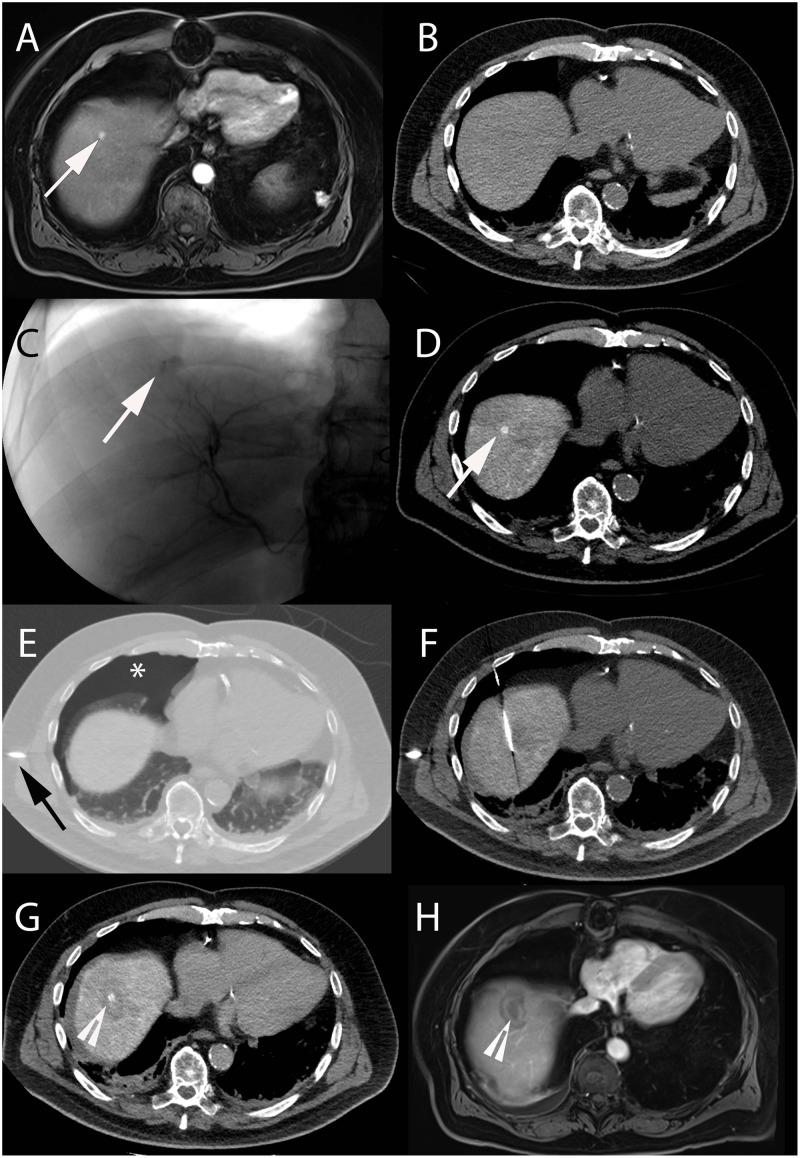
Intra-arterial hepatic administration of Lipiodol for tumor marking and visualization before radiofrequency ablation in a 64-year-old male with a history of alcoholic cirrhosis and HCC. The tumor lesion (arrow) easily identified on contrast-enhanced arterial phase T1-weighted MRI (A) was neither visible on ultrasound nor on unenhanced (B) and contrast-enhanced (not shown) CT images. (C) Selective angiography from the right hepatic artery demonstrated an area of contrast blush corresponding to the HCC lesion (arrow). (D) Unenhanced CT-scan performed immediately after Lipiodol injection from the right hepatic artery showed avid Lipiodol uptake by the tumor that became visible on CT (arrow). (E) Iatrogenic CO2 pneumothorax (^∗^) was performed using a Veress needle (black arrow) to allow lesion targeting without touching the lung. (F) The radiofrequency probe could then be precisely placed into the lesion using CT-guidance. (G) Post-ablation unenhanced CT showed hypodense area surrounding the tumor with satisfactory ablation margins (arrowhead). (H) Contrast-enhanced arterial phase T1-weighted MRI performed 1-month after treatment showed complete tumor ablation (arrowhead).
